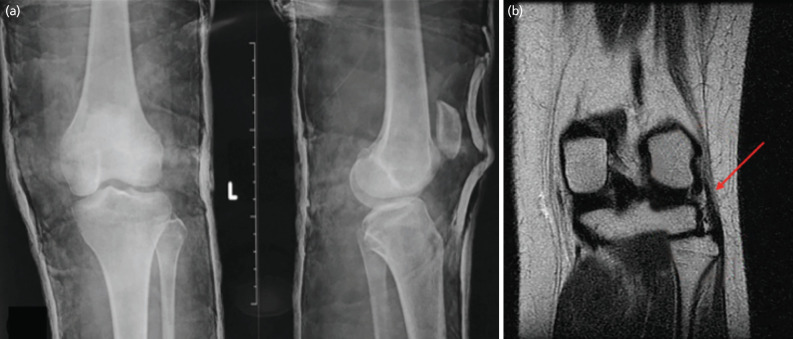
(a) Post-reduction radiograph. (b) MRI showed partial discontinuity of the left lateral collateral ligament and joint effusion. No osteochondral defect or loose bodies (shown by the red arrow) existed.
